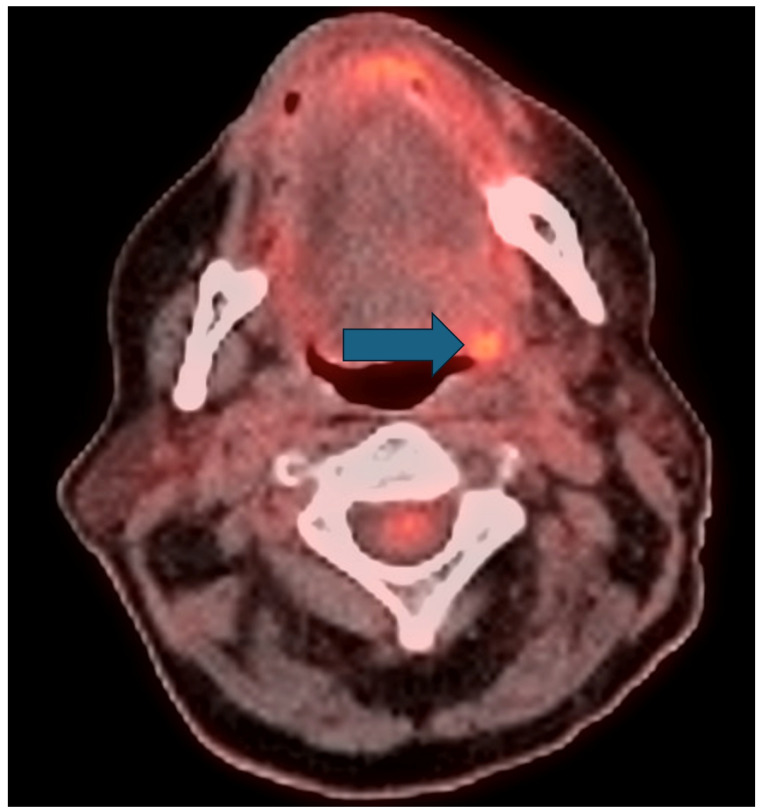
Axial fused image of a post-therapy FDG PET/CT in a 63-year-old woman with left tonsillar squamous cell carcinoma which demonstrates potentially ambiguous tongue uptake at the site of the primary tumor (uptake between that of the blood pool and the liver marked with arrow) that may result in a variable interpretation if written in free-form text. For this patient, this was the only residual site of uptake, thus determining her post-therapy disease status. Indeed, even by SICs there is variability in the resulting clinical assessment with both negative and equivocal disease assessments possible in this case. More specifically, this finding is assigned as PR by PERCIST given the large and incomplete decline in uptake; H2 (equivocal) by Hopkins, being between the IJV and the liver; NIRADS 2 (equivocal), having low-grade focal uptake; M2 (equivocal), being between the mediastinal blood pool and the liver; C 2 (negative) by Cuneo since the uptake is between the mediastinal blood pool and the liver but the background is less than the reference lesion. Porceddu does not evaluate the primary site and thus is not applicable for this exam.
